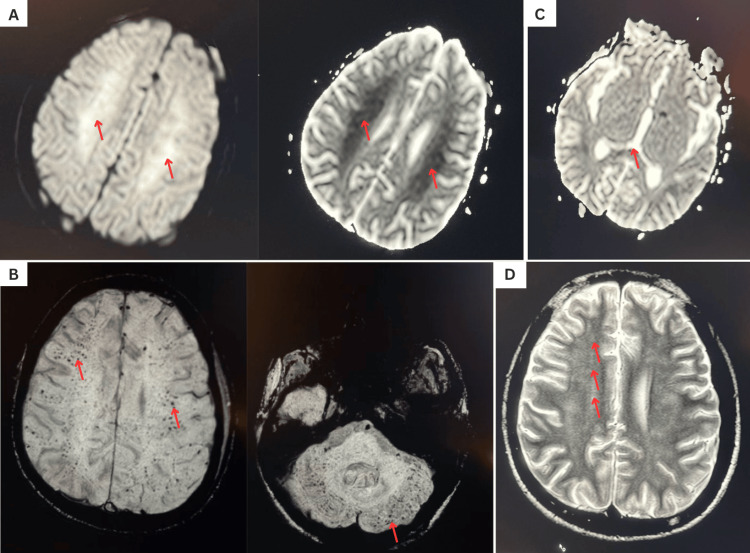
Magnetic resonance imaging findings consistent with cerebral fat embolization syndrome (A) Diffusion-weighted imaging demonstrating diffusion restriction within the bilateral corona radiata.
